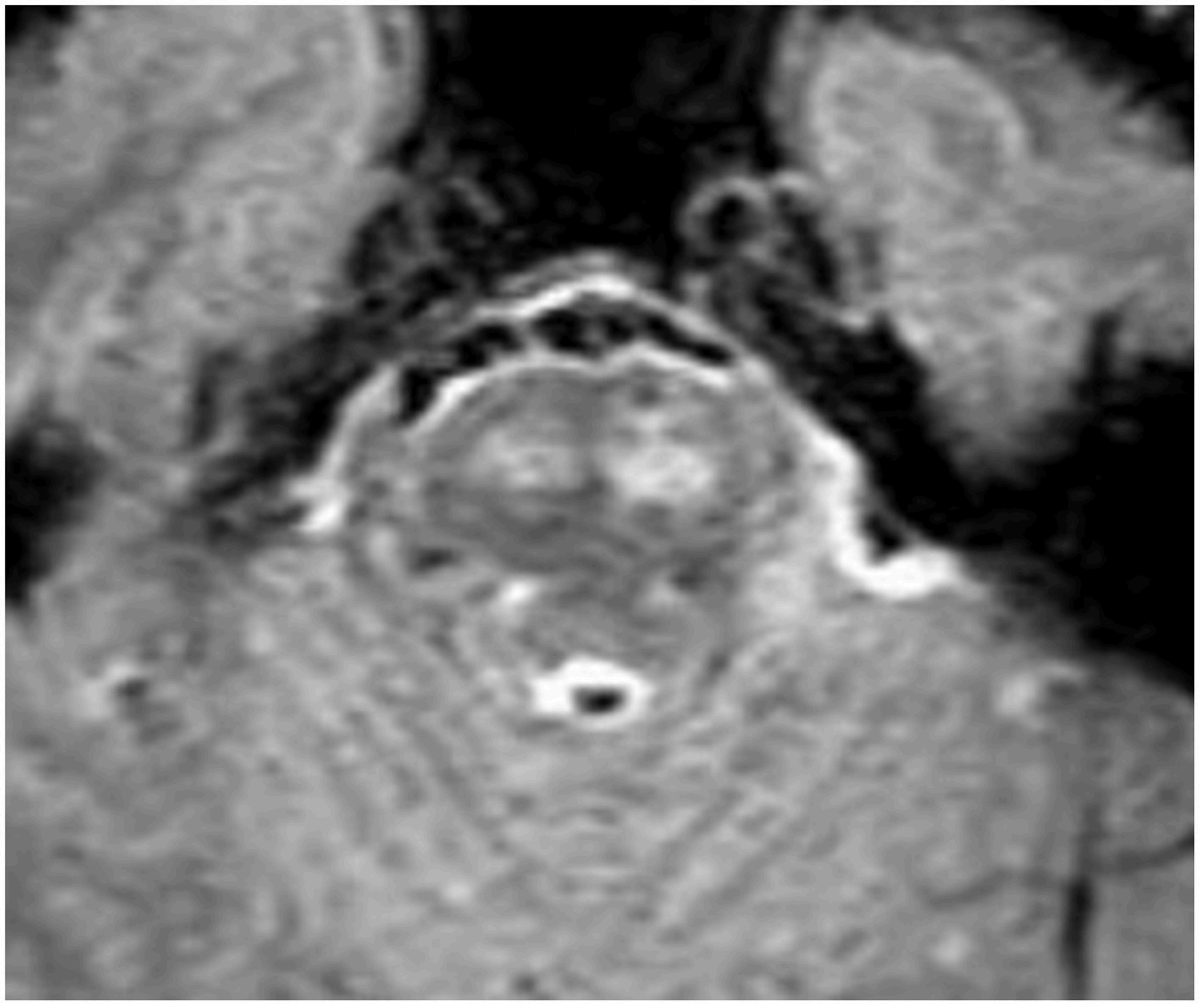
Fluid attenuated inversion recovery (FLAIR) demonstrating pontine hyperintensity from lymphoma involvement.
